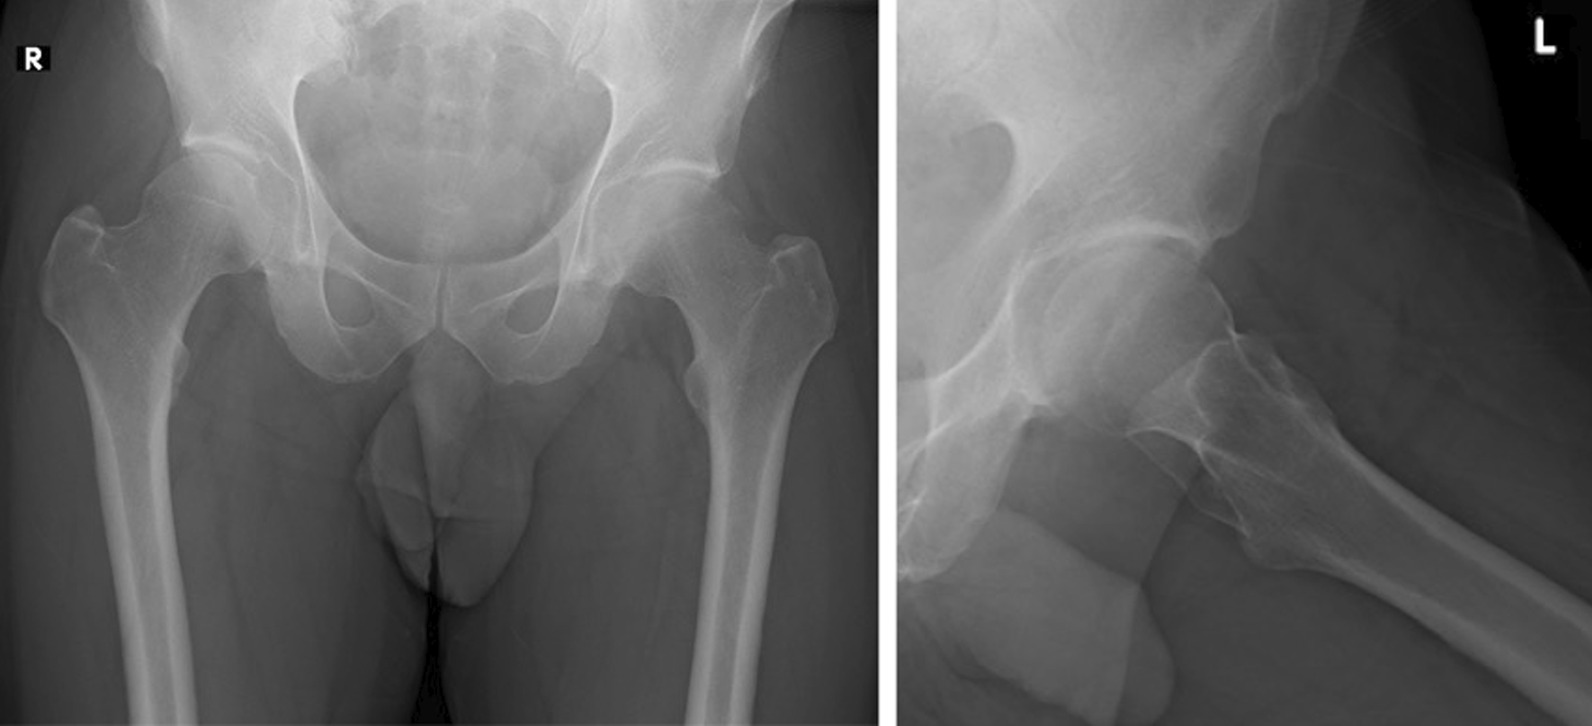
Plain radiography images taken 3 months after the injury. Anteroposterior view (left) and Lauenstein view (right) of the hip joint did not show any obvious abnormal findings
